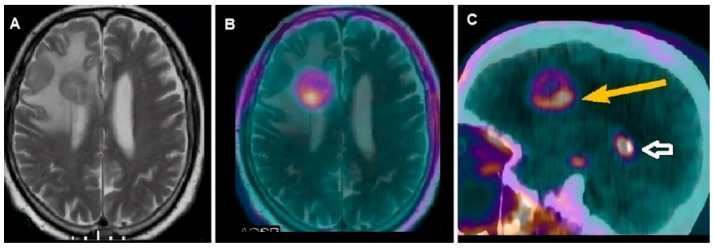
16-year-old male patient affected by astrocytoma, treated with radiotherapy and suspected for relapse at Magnetic Resonance Imaging (MRI) examination (A). Off-line co-registered [^18^F] choline PET/MRI (B) axial slice well demonstrated intense tracer in the peripheral and posterior part of the large morphological lesion detected by MRI, thus confirming the diagnosis of recurrent tumor. In (C), sagittal fused PET/CT slice well-depicted tracer uptake in the tumor (yellow arrow), also showing the physiological uptake in the choroid plexus (white bordered arrow).
